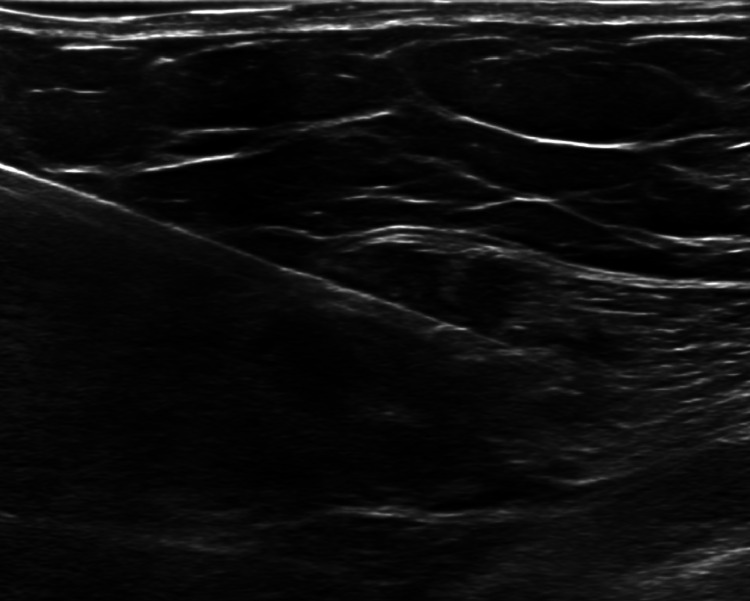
Image from the patient's ultrasound-guided biopsy of the mass within the right pectoralis muscle.
